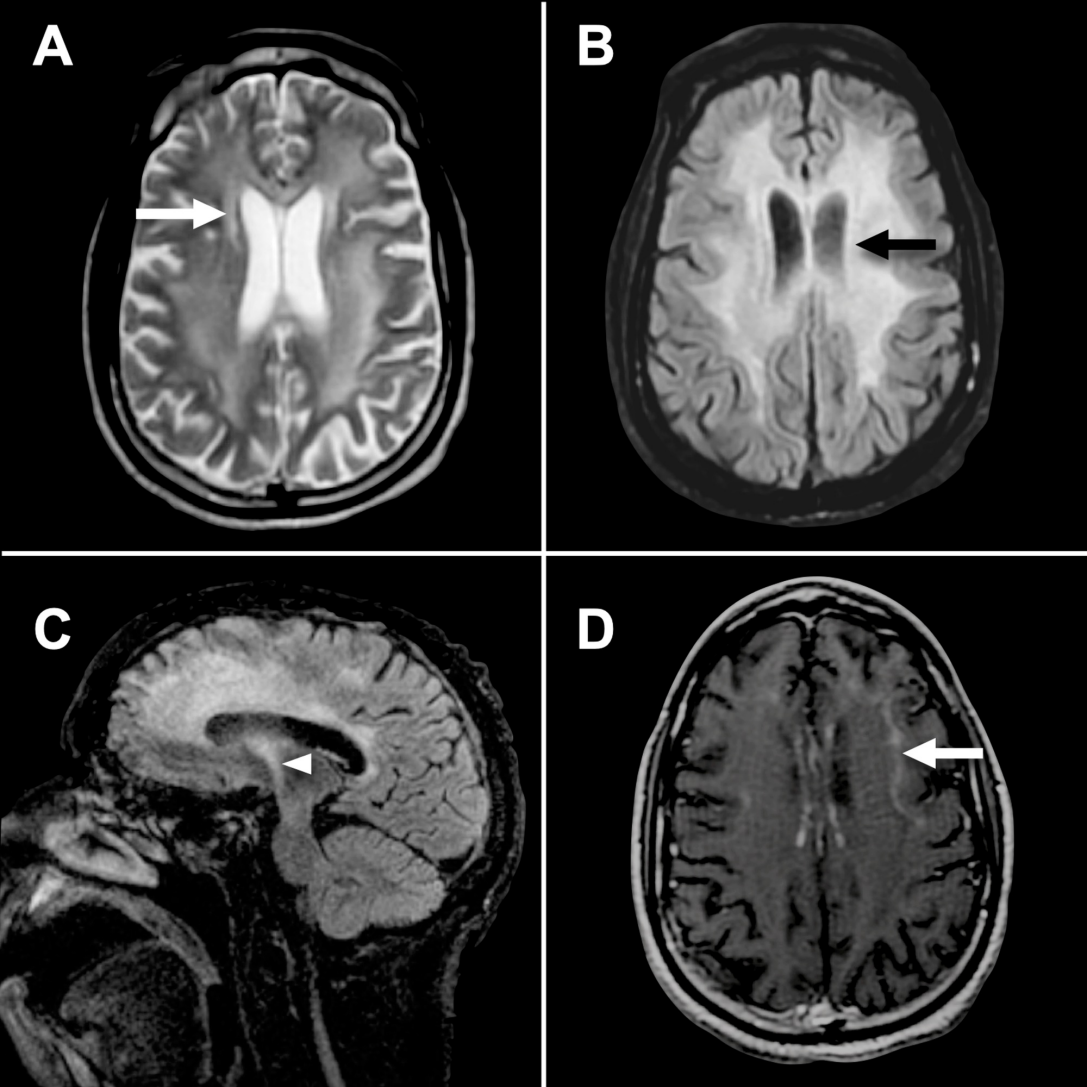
Follow-up multisequence magnetic resonance (MR) imaging of the brain with gadolinium contrast after a period of 60 days from initial MRI. (A) Axial T2 weighted sequence showing confluent areas of the T2 hyperintensity in the white matter bilaterally, with additional deep white matter involvement on follow-up (arrow). (B) T2 FLAIR sequence showing T2 hyperintense changes in the periventricular region without improvement compared to the prior study (arrow). (C) Sagittal T2 FLAIR sequence shows new regions of T2 hyperintense signal extending inferiorly along the corticospinal tract (arrowhead), predominantly on the left. (D) Axial T1 weighted post-contrast sequence demonstrating linear region of peripheral contrast enhancement (arrow).
